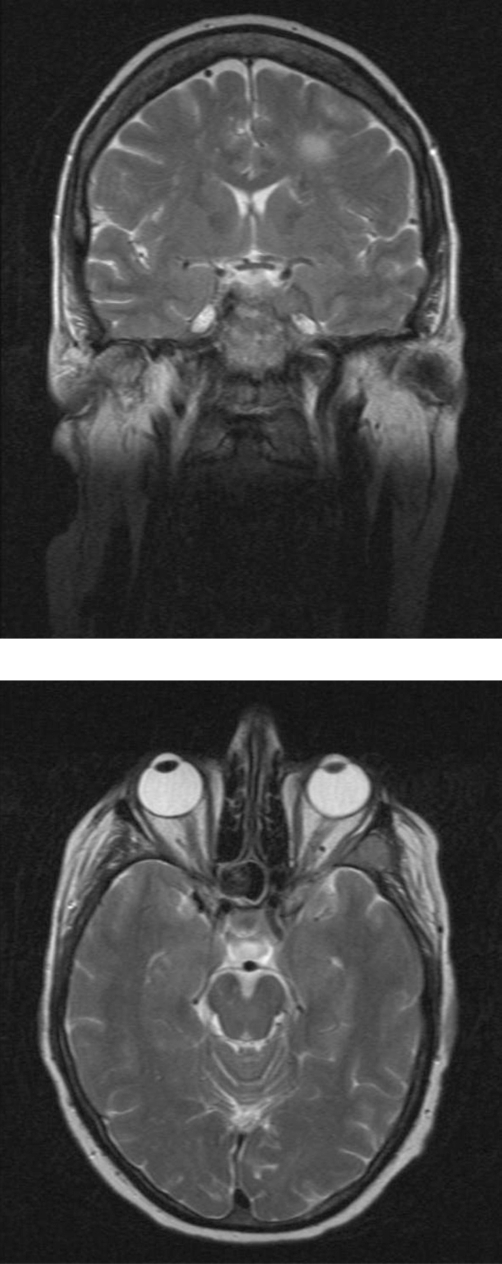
Magnetic resonance imaging of patient’s brain shortly after admission. Gadolinium enhanced Magnetic Resonance Image demonstrating two enhancing meningeal-based lesions one in the left frontal extra-axial region, and the second lesion on the left side of the cavernous sinus. The lesion in the left cavernous sinus is also associated with bone destruction. Given this patients past medical history, these images are consistent with extra axial soft tissue deposits of myeloma.
